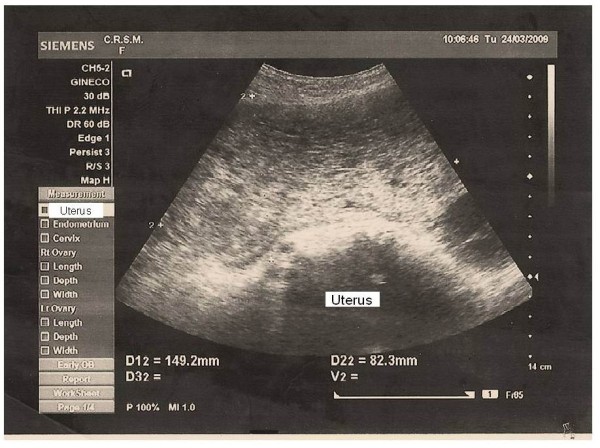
Typical sonographic appearance of hydatidiform mole (case 2).
